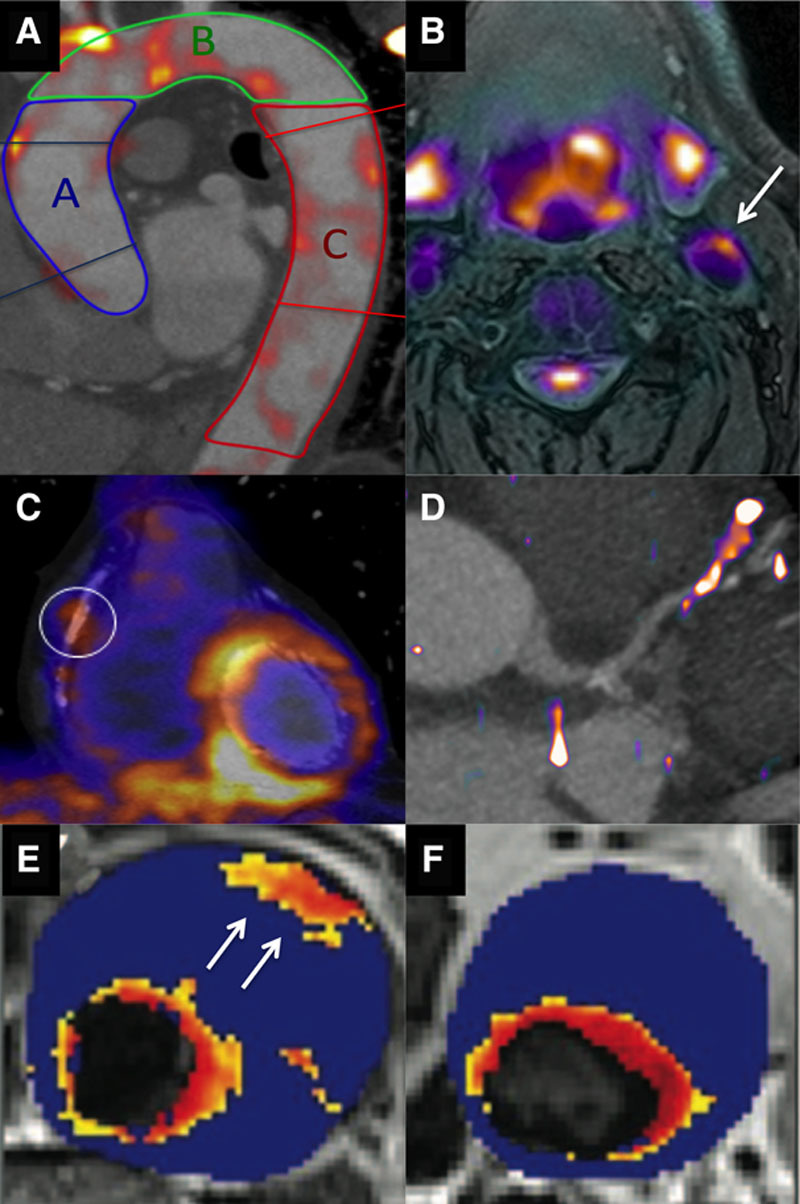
Molecular imaging of vascular inflammation activity. A, 18F-fluorodeoxyglucose (18F-FDG) positron emission tomography (PET) image fused with contrast computed tomographic (CT) angiogram of the thoracic aorta, demonstrating regions of increased activity in the ascending aorta (blue), aortic arch (green), and descending aorta (red). B, 18F-FDG PET/magnetic resonance (MR) image of the carotid arteries demonstrating increased activity in the left carotid artery and the excellent soft tissue contrast provided by MR. C, 18-FDG PET fused with a CT coronary angiogram demonstrating increased uptake in the left ventricle but also in a remote plaque in the mid right coronary artery. Image in panel C reproduced from Cheng et al^21^ with permission of the publisher. Copyright © 2012, the Society of Nuclear Medicine and Molecular Imaging, Inc. D, 68Ga-DOTATATE PET/CT image with increased activity localizing to a plaque in the mid left anterior descending artery. E, T2* Map from patients with an abdominal aortic aneurysm that had been administered ultra small particles of iron oxide (USPIO). A hot spot is observed in the anterior wall of the aneurysm (arrow). F, In a second patient, focal areas of increased USPIO uptake can be observed (the increased signal adjacent to the lumen is considered normal because of high signal in the blood pool). Images in panels E and F reproduced from Richards et al^23^ with permission of the publisher. Copyright © 2011, Wolters Kluwer Health, Inc.
